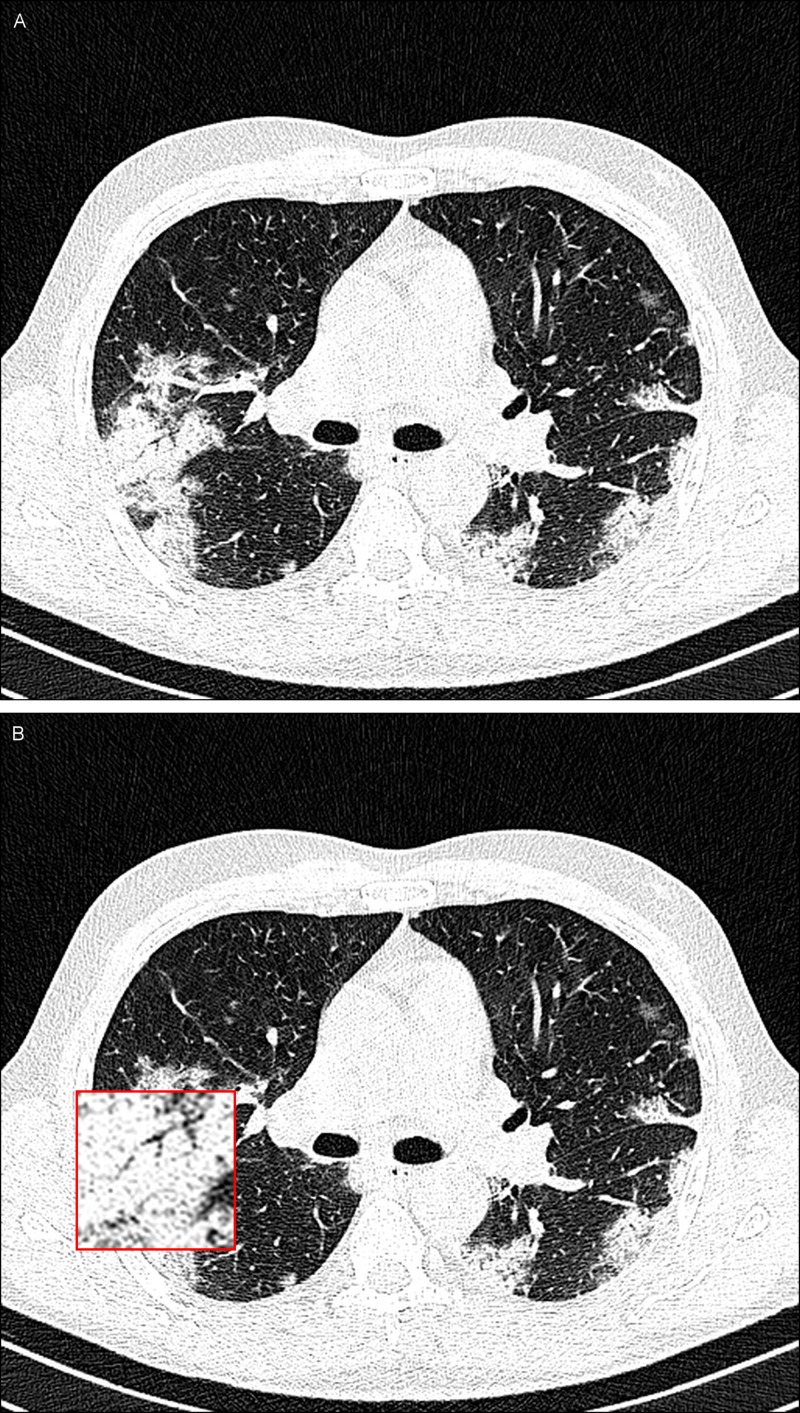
A 43-year-old man with COVID-19. A noncontrast-enhanced CT image of the lungs showing consolidation in both lungs (A) and an inset magnified view of the lesion for better delineation at the location of consolidation in the right lower lobe of the lung (B).
